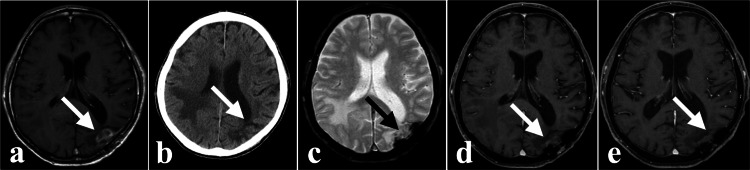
Time course of the left occipital lobe lesion. (a), (b), and (c) are pre-CKR images taken 3 months after brain surgery. (a), (d), and (e) show enhanced MRIs. (a) Enhanced lesion (indicated by the white arrow). (b) No rebleeding visible on plain CT (indicated by the white arrow). (c) No rebleeding visible on T2*WI (indicated by the black arrow). (d) Shrinkage of the enhanced lesion observed 1 month after CKR (indicated by the white arrow). (e) No lesion recurrence observed 12 months after CKR (indicated by the white arrow). CKR: CyberKnife radiotherapy; T2*WI: T2*-weighted image.
